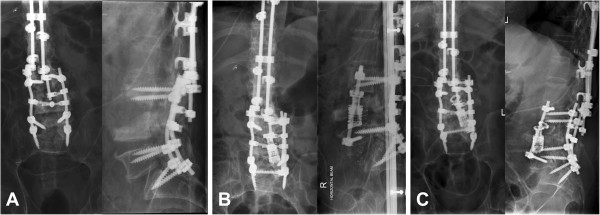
Plain radiographs following the first and second surgeries showing posterior extension of instrumentation to the sacrum with pedicle screws and rods, anterior reconstruction with an expandable titanium cage and anterior rod and screws, and subsequent disengagement of the cage and shifting of the posterior instrumentation. (A) Anteroposterior and lateral radiographs taken after the first surgery showing posterior extension of instrumentation from L3 to S1 with pedicle screws and rods. (B) Radiographs taken immediately after the second surgery involving L4 corpectomy and reconstruction with an expandable titanium cage and anterior rod and screws. (C) Radiographs taken after the patient was allowed to mobilize, showing disengagement of the cage and shifting of the posterior instrumentation.
